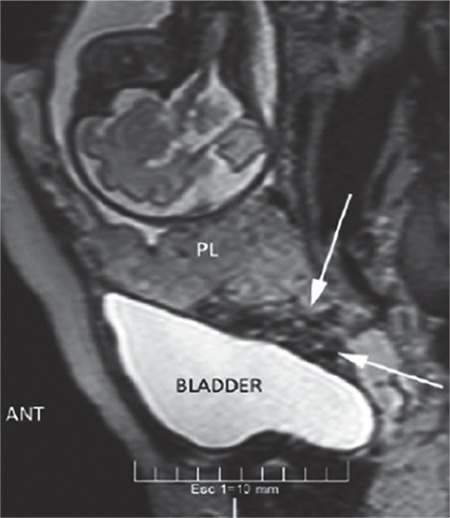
Magnetic resonance imaging of placenta percreta bladder invasion
